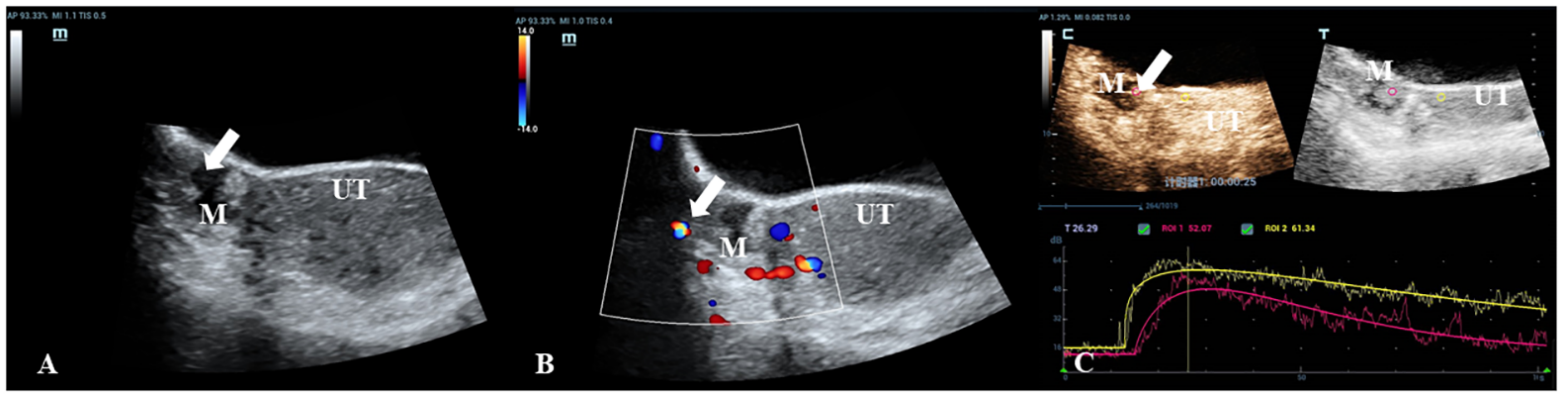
A 30-year-old woman with a pathologically proven borderline cystadenoma. (A) Transabdominal gray-scale ultrasound shows an 18-mm cystic mass (M) with a small 13 x 8mm papillary component at the right of uterus (UT). (B) Color Doppler reveals no blood flow signal in the papillary portion (color score=1). (C) CEU curve demonstrates that the tumor showed hypoenhancement in initial perfusion and faster washout compared with myometrium (mass: red, myometrium: yellow).
